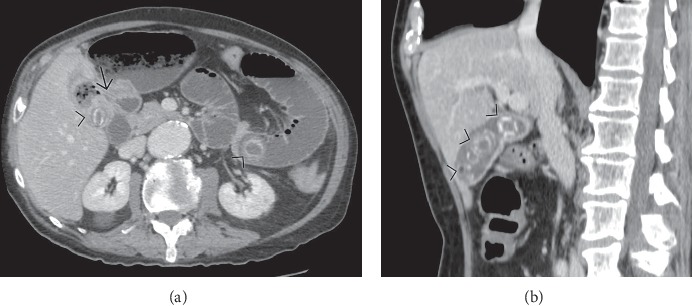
Contrast-enhanced computed tomography (CT) in a patient with gallstone ileus: two peripherally calcified stones (arrowheads in (a)) are present, the first visible in the gallbladder and the latter enlodged in the jejunum; the fistulous tract between the gallbladder and the duodenum is well visualized (arrow). A sagittal reformatted CT image obtained a few years before shows the gallbladder lumen filled with multiples stones (b).
